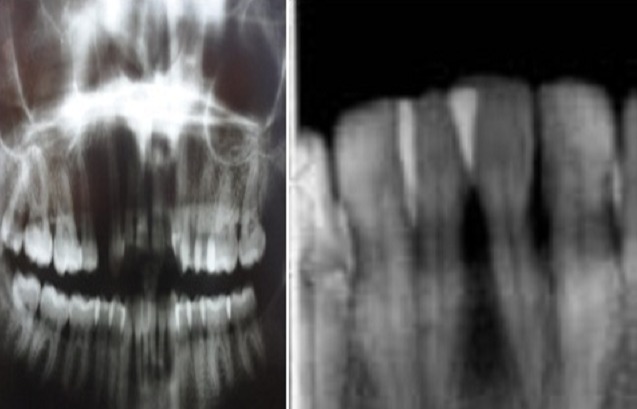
Les radiographies-X standards (incidences panoramique et retro dentaire): signes de parodontite
